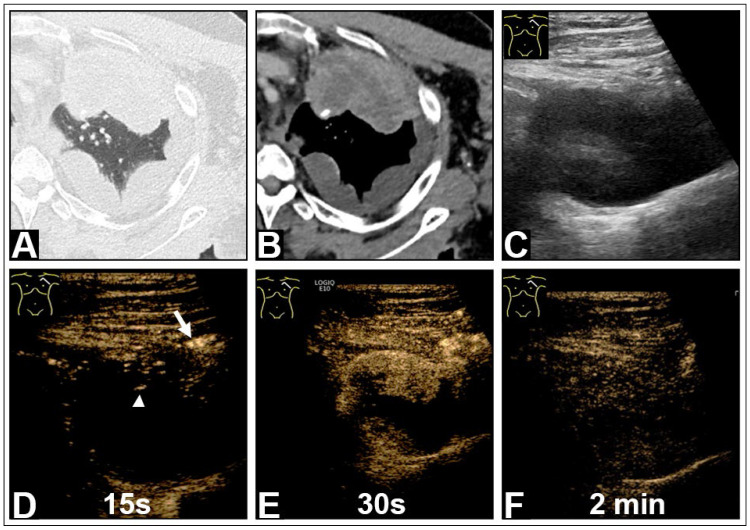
63-year-old male patient with a known diagnosis of undifferentiated pleomorphic sarcoma and multiple pulmonary lesions on CT (A,B). On B-mode ultrasound (C), a hypoechoic pleura-adjacent pulmonary nodule was identified. On CEUS (D), the lesion (arrowhead) demonstrated a bronchial-arterial enhancement pattern, with enhancement occurring simultaneously with the arrival of contrast in the intercostal artery (arrows). The lesion exhibited marked and inhomogeneous enhancement (E), followed by an early decrease in enhancement at approximately 2 min (F), consistent with rapid washout.
